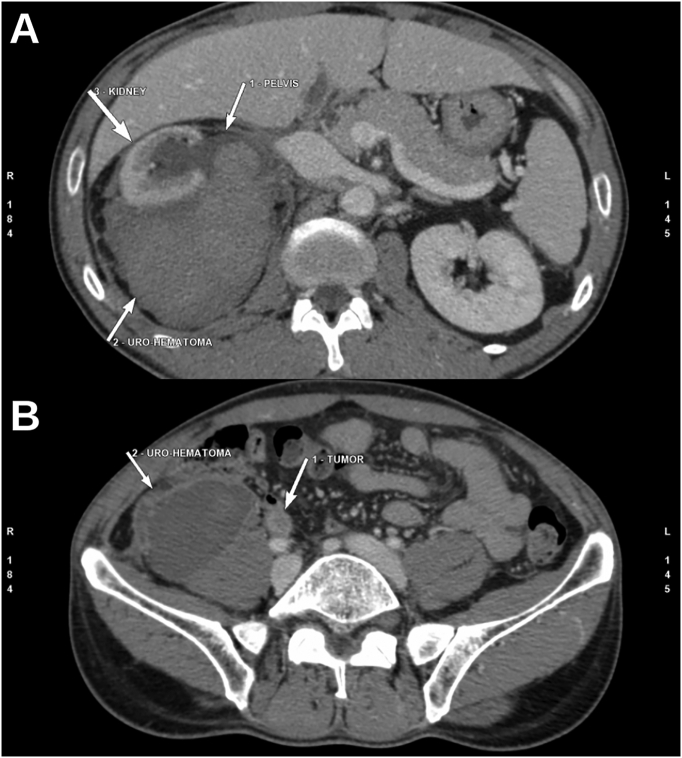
Abdominal CT images at the parenchymal phase: (A) A retrorenal collection showing a mixed liquid and blood densities; (B) The retrorenal collection is extending to pelvic fossa with the visualization of an enhancing lesion at the lumbar ureter.
